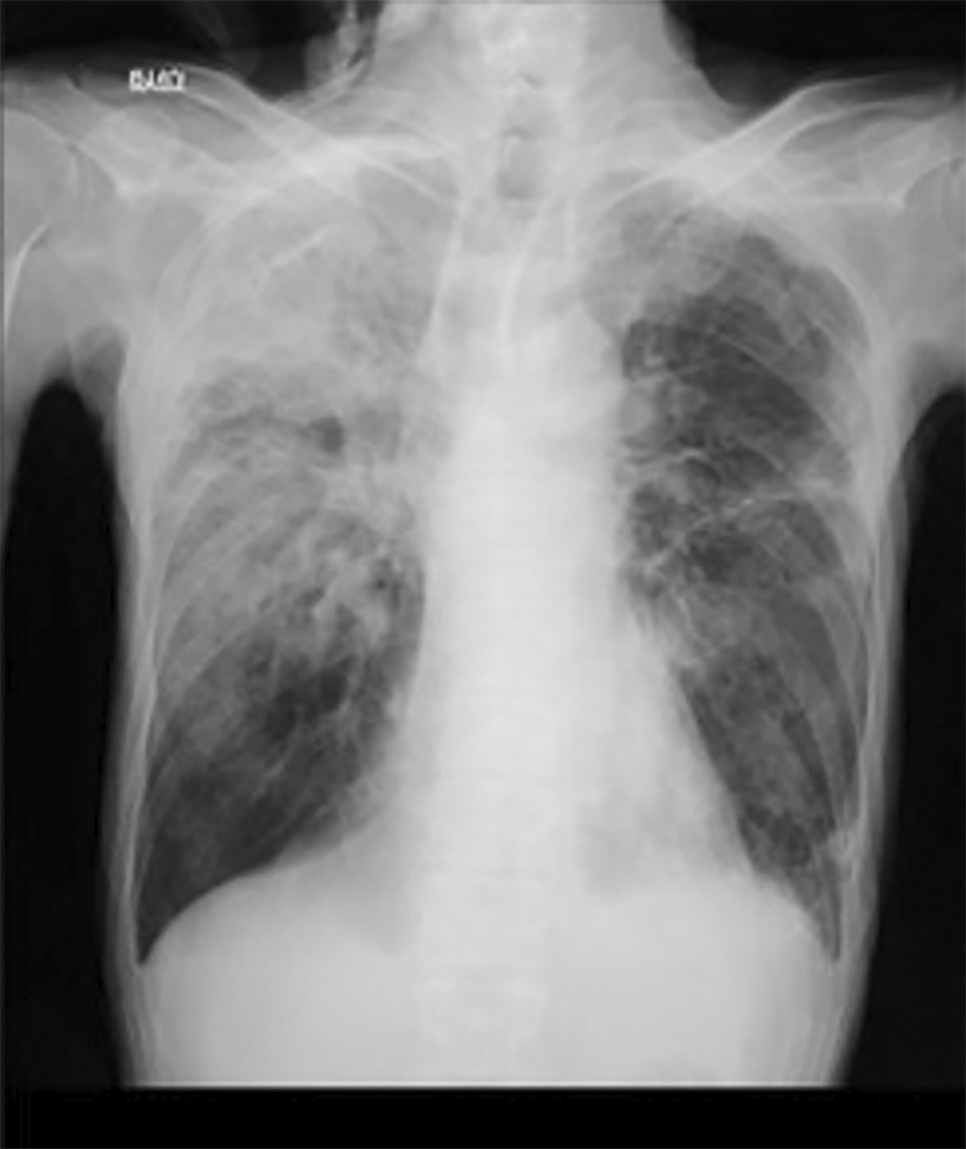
Chest radiography at initial examination. Chest radiography showing an infiltrative shadow in the right lung field, raising suspicion of pneumonia and prompting referral for further evaluation.
